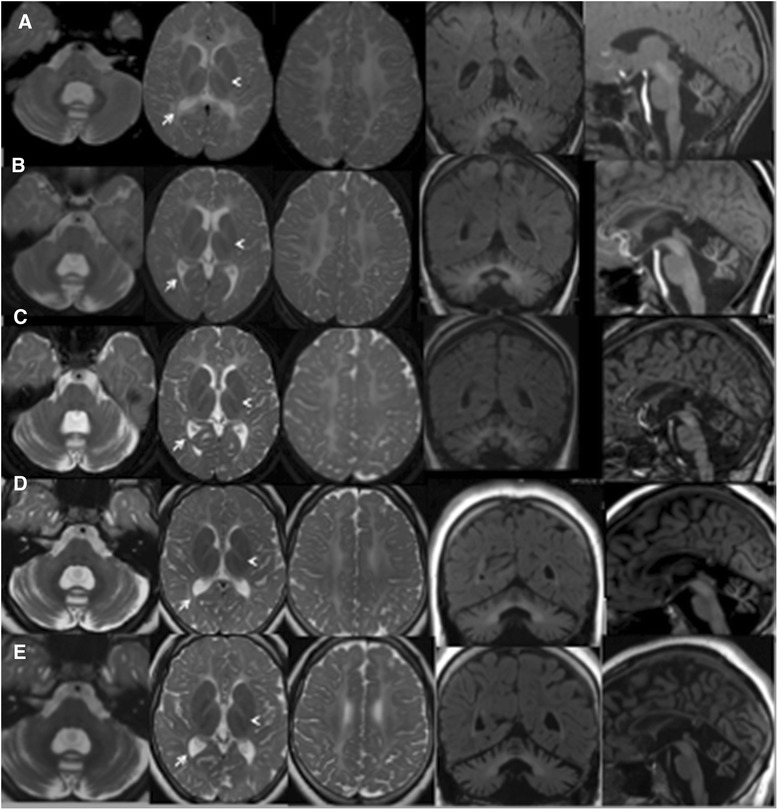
Hypomyelination with cerebellar atrophy: long term follow-up evaluation. a (7 years). b (10 year). c (13 years). d (15 years). e (19 years): Axial and coronal T2-weighted images show extensive cerebral white matter (WM) abnormalities with predominant involvement of the deep and subcortical WM; note the sparing of optic radiation (arrows), perirolandic WM and partial splenum corpus callosum. The head arrows (a-e), instead, indicate small hypointense dot in the posterior limb of the internal capsule. Mild abnormal hyperintensity involves the cerebellar WM. Sagittal T1-weighted images show a thin corpus callosum and shrunken cerebellar cortex with enlarged fissures. The pons is normal. At age of 13 years MRI (c) revealed a mildly increased cortical atrophy of cerebellar hemispheres that remained stable in the following MRI exams. No significative changes were observed during the yrs on WM abnormalies
